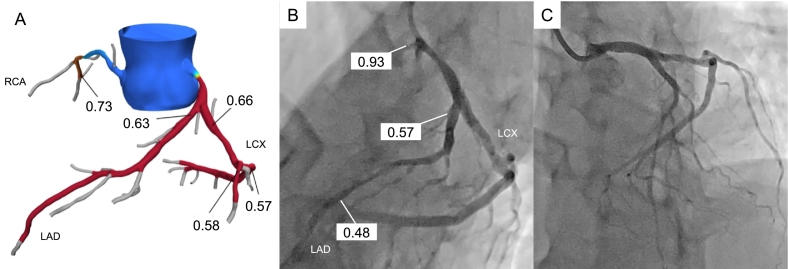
Coronary artery findings in FFR-CT, invasive FFR, and post percutaneous coronary intervention for the LMCA. (A) Fractional flow reserve from computed tomography (FFR-CT) showed hemodynamically significant stenosis in the left main coronary artery (LMCA). (B) The invasive fractional flow reserve (FFR) value decreased to 0.57 at distal site of the LMCA. (C) Invasive coronary angiography after deployment of a drug-eluting stent in the LMCA-LAD. LAD, left anterior descending; LCX, left coronary circumflex; RCA, right coronary artery.
